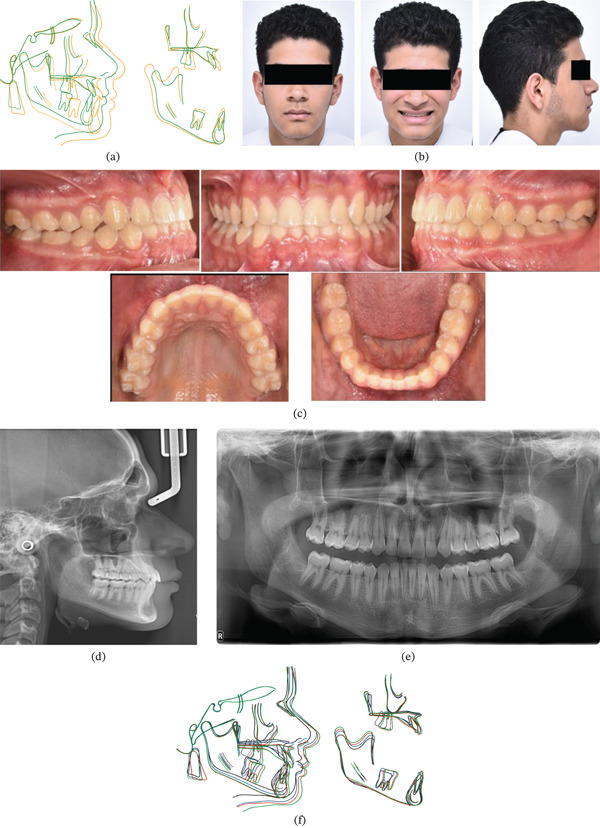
(a) Cephalometric superimposition of active treatment changes. (b) Long‐term (9‐year) follow‐up facial photographs showing maintained results. (c) Long‐term intraoral photographs demonstrating stable occlusal relationships. (d) Long‐term panoramic radiograph confirming periodontal health and root parallelism. (e) Long‐term lateral cephalometric radiograph showing postpubertal growth pattern. (f) Comprehensive cephalometric superimposition tracing growth changes through postpubertal development.
